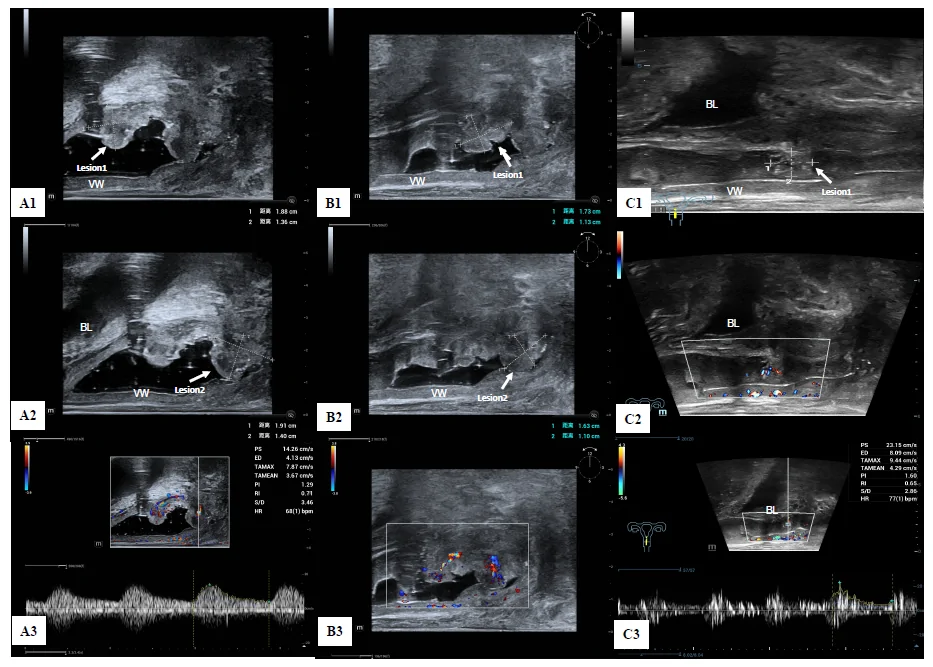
Transrectal high-frequency ultrasound images. (A1): Two-dimensional ultrasound image and size measurement of vaginal Lesion 1 before treatment.(A2): Two-dimensional ultrasound image and size measurement of vaginal Lesion 2 before treatment.(A3): Blood flow image and spectral Doppler measurement of the vaginal Lesion before treatment.(B1): Two-dimensional ultrasound image and size measurement of vaginal Lesion 1 after external beam radiotherapy.(B2): Two-dimensional ultrasound image and size measurement of vaginal Lesion 2 after external beam radiotherapy.(B3): Blood flow image of the vaginal Lesion after external beam radiotherapy. (C1): Two-dimensional ultrasound image and size measurement of the vaginal Lesion six months after treatment.(C2): Blood flow image of the vaginal Lesion six months after treatment.(C3): Spectral Doppler measurement of the vaginal Lesion six months after treatment. BL=bladder, VW=vaginal wall. Flow direction Red: toward transducer, Blue: away from transducer.
